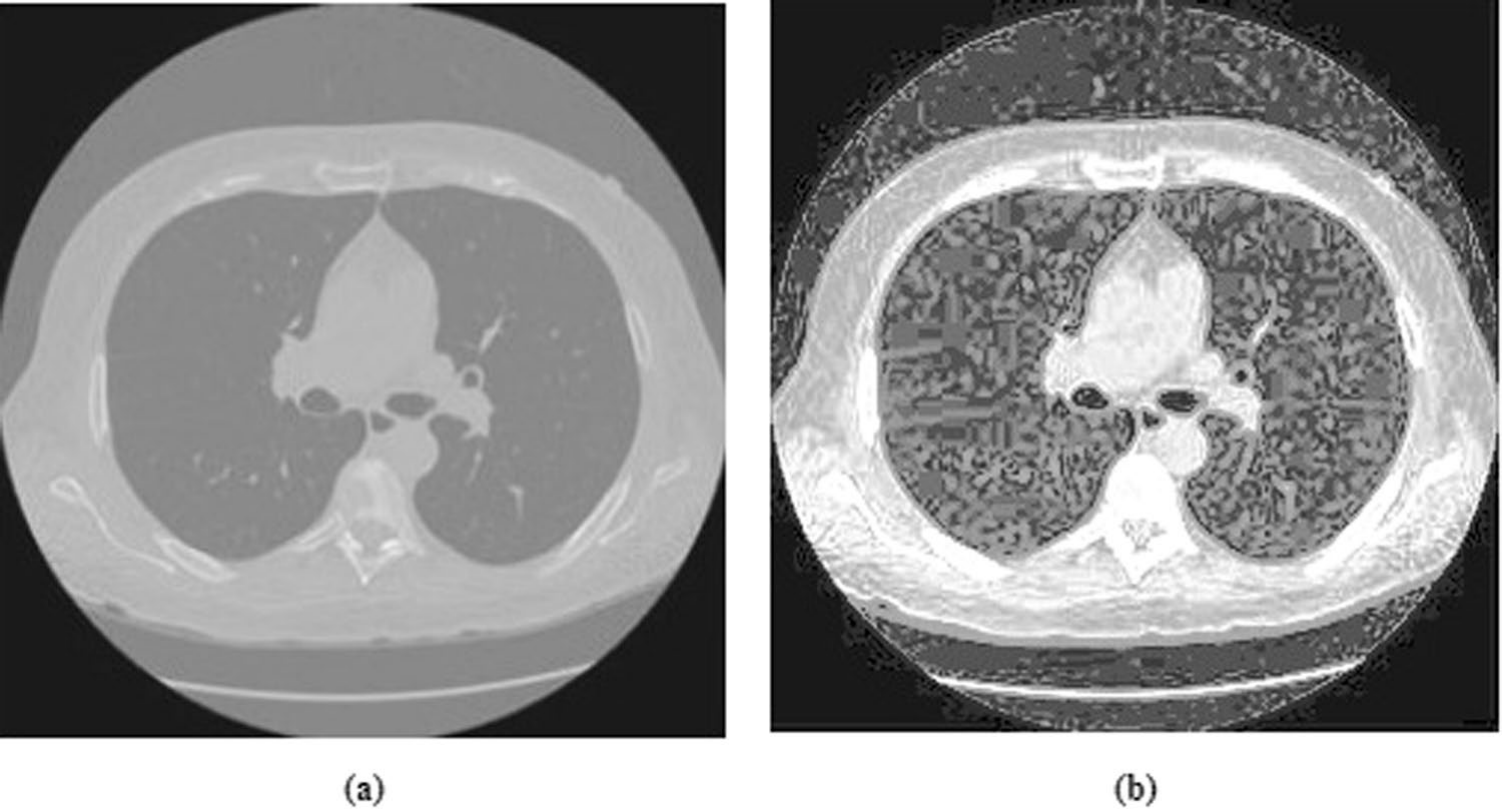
CT image contrast enhancement results. (a) Before contrast enhancement. (b) After contrast enhancement.
